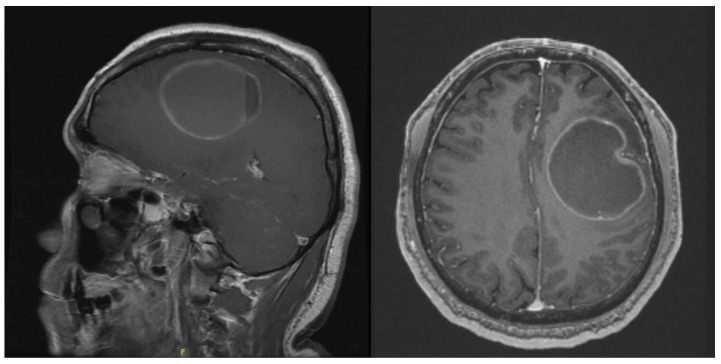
Contrasted T1 MRI showing the intra-axial cystic tumour.
